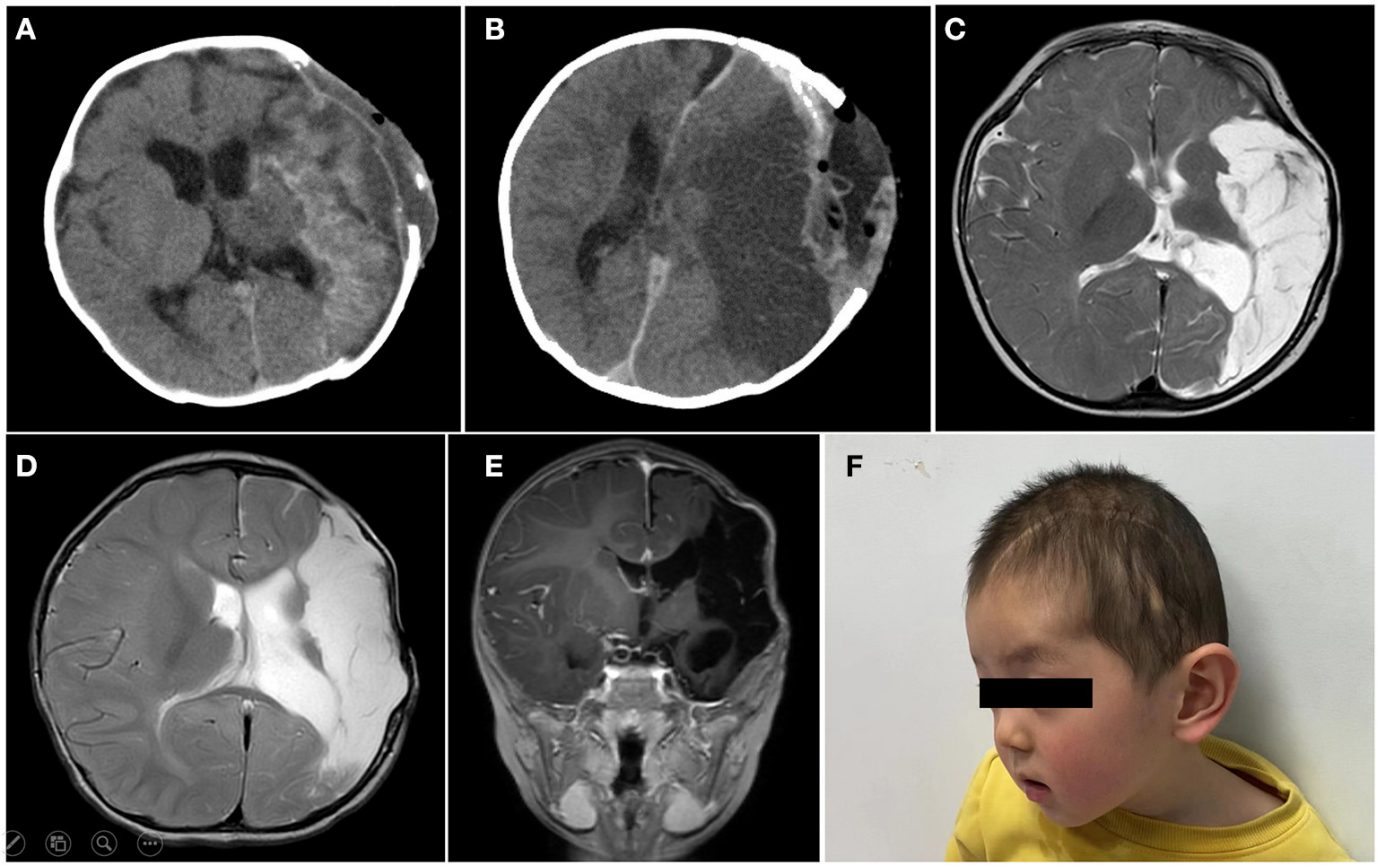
On postoperative-day (POD) 3 (A) and POD 14 (B), head CT re-examination revealed no tumor residues. The brain MR examination of 6 months (C), 12 months (D,E) postoperatively, suggested ischemic and liquefaction changes in the part of left frontal and temporal lobes and basal ganglia. No local recurrence or metastasis was observed. (F) Briefly, 12 months postoperatively, a skull defect can be seen in the forehead of the patient, and the spontaneous facial expressions are symmetrical but involuntary salivation was observed.
